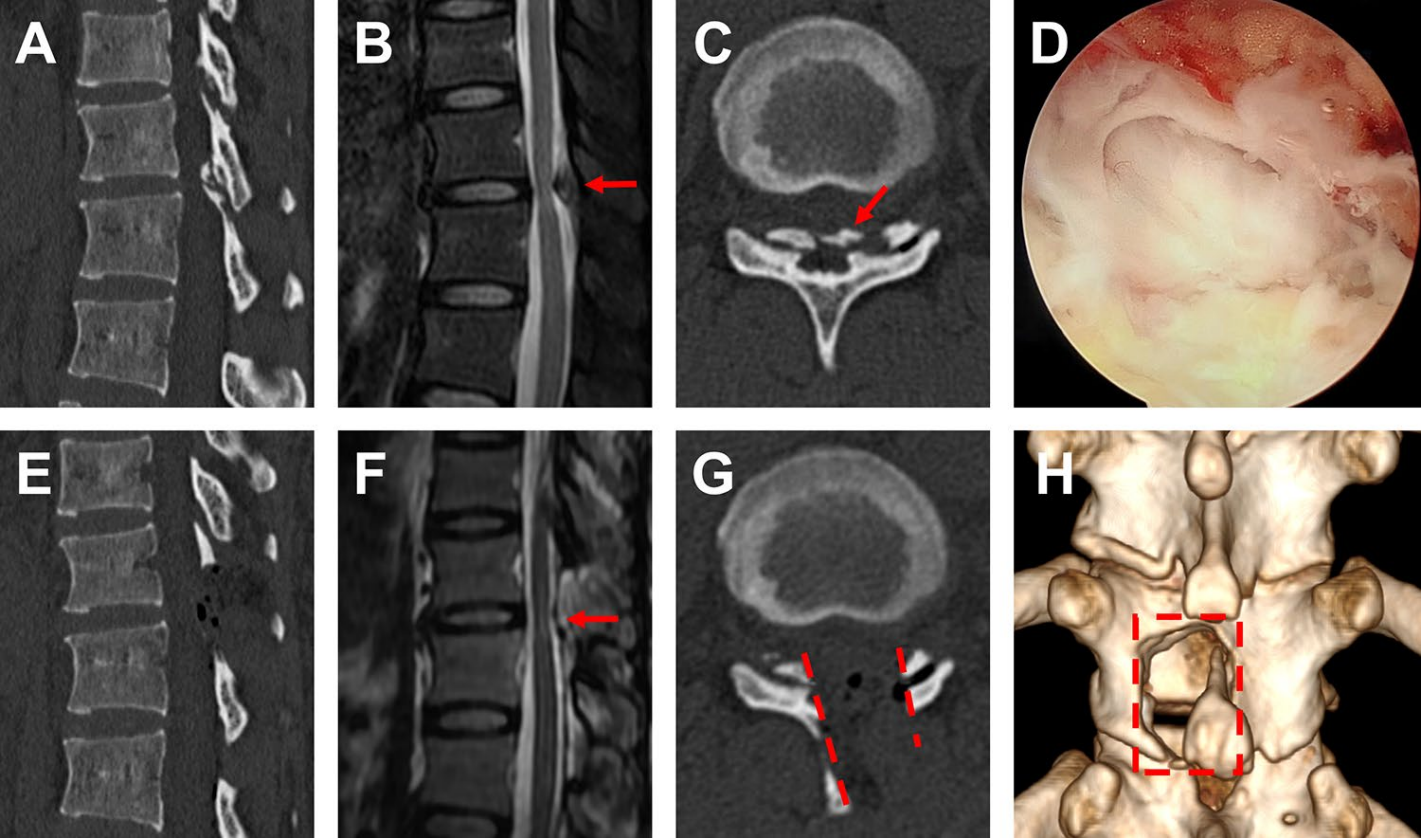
DO with TOLF treated with OSE procedure. (A–C) Preoperative sagittal, axial CT and MRI images of TOLF, and the ossification of dura mater compressed the spinal cord at T11/12 (As shown by the red arrow). (D) The view of spinal cord during operation after removing the ossification of dura mater on endoscope. (E–G) Postoperative sagittal, axial CT and MRI images, demonstrated a complete remove of ossification lesion originating from dura mater and ligamentum flavum (As shown by the red arrow and dashed). (H) Postoperative 3D-CT image showed the range of unilateral laminectomy at T11/12 (As shown in the red dashed region), bilateral decompression of spinal cord through unilateral approach.
